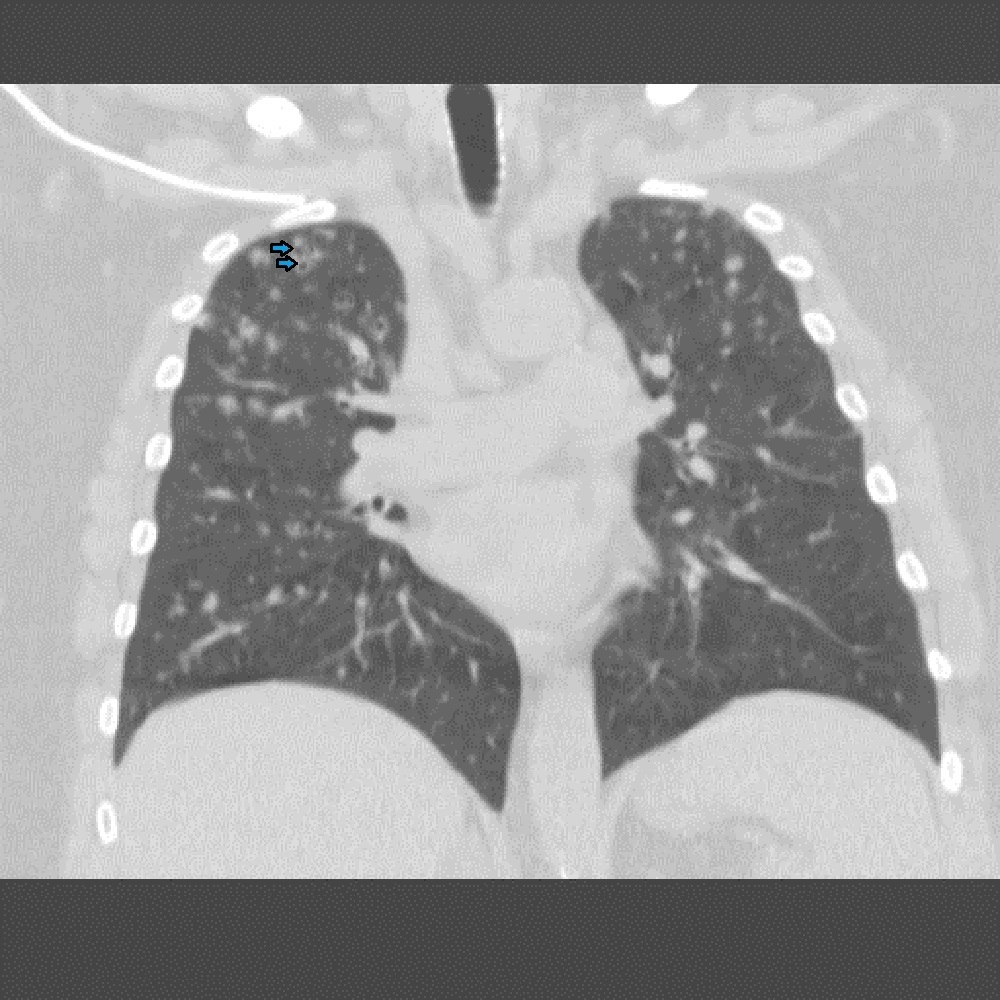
Computed tomography of the chest on initial evaluation demonstrating subcentimeter cavitary nodular lesions
